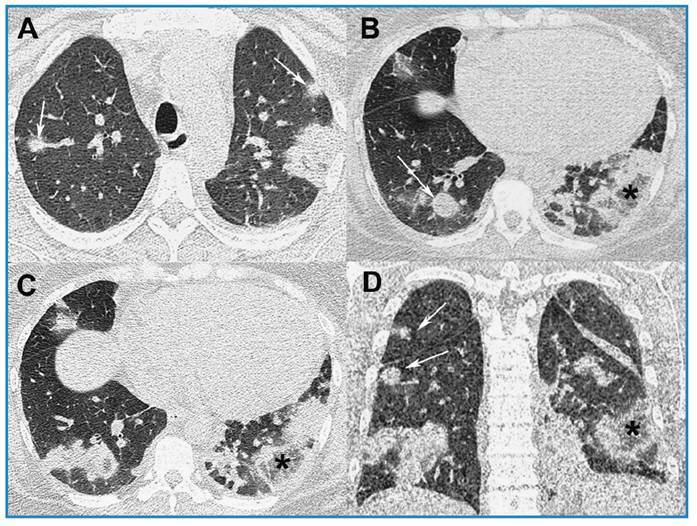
Chest computed tomography images with axial (A-C) and coronal (D) reconstruction showing multiple ill-defined nodules in both lungs (arrows) and areas of ground-glass opacities and consolidations, some forming reversed halo signs (asterisks).
